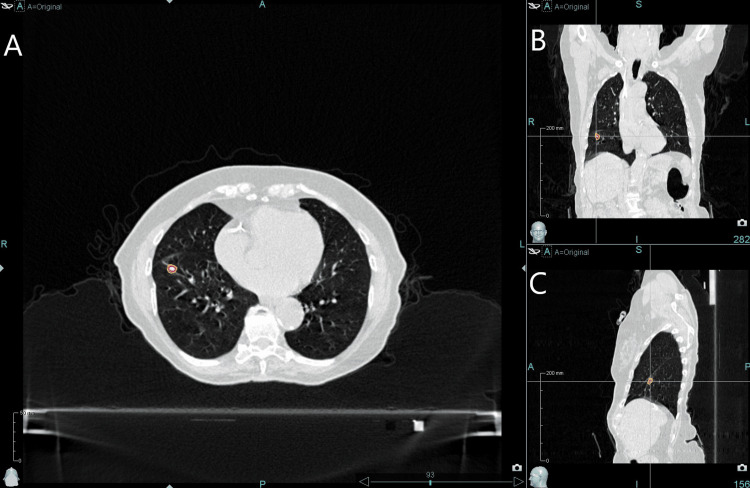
Patient's planning CT image with TTV and GTV indicated by red and orange contours, respectively, in axial (A), coronal (B), and sagittal (C) views. The image window width and level were set to the lung preset CT: computed tomography; TTV: tracking target volume; GTV: gross tumor volume
